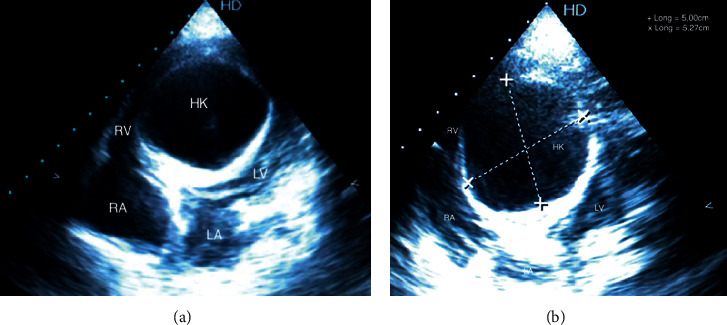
Echocardiography: transthoracic apical 4-chamber view showing a large cystic mass splitting the interventricular septum (50 mm/52 mm). HK: hydatid cyst, LV: left ventricle, LA: left atrium, RV: right ventricle, RA: right atrium.
